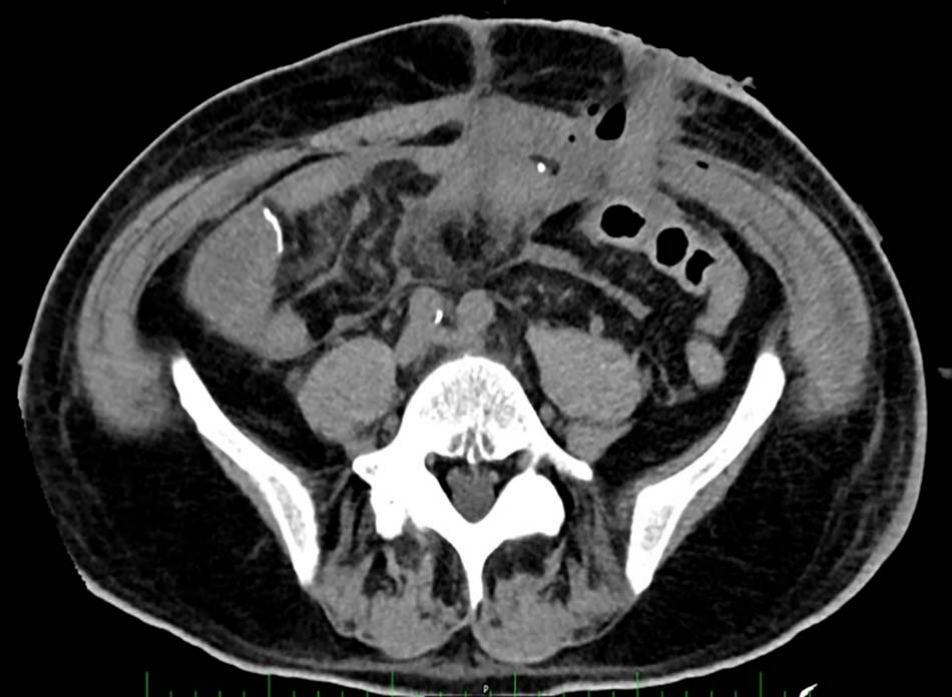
Post-operative Day 14. Abdominal CT-scan with free intraperitoneal fluid, without sign of ileus, perforation or anastomosis release.
